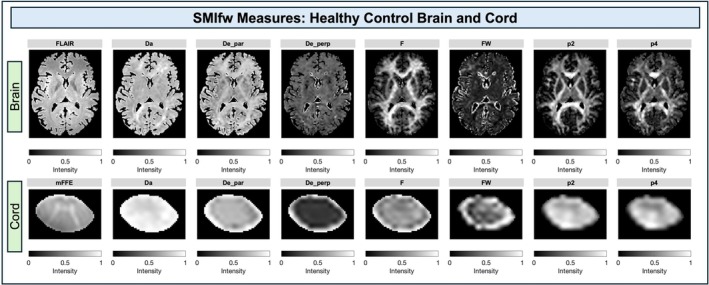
All measures captured by in the SMIfw diffusion model for one HC (28.9‐year‐old female) in the brain and SC. The T2 FLAIR and mFFE images were normalized between 0 and 1 for visualization and included as anatomical references. For diffusion metrics, intensity values within brain and cord regions were independently normalized by their respective 99th percentile values to scale diffusion maps between 0 and 1 for visualization.
